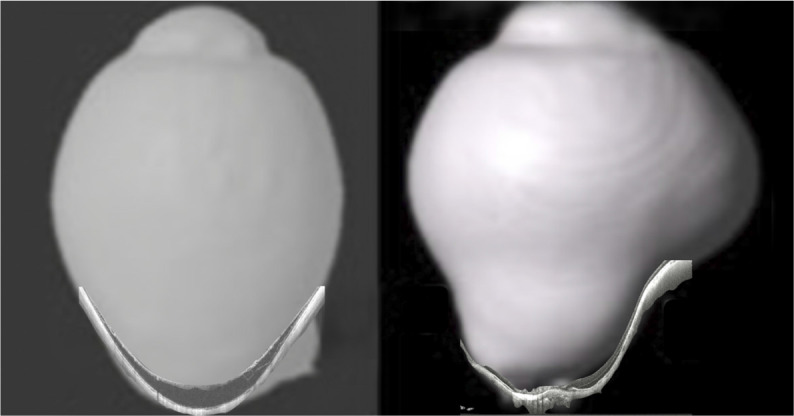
3D magnetic resonance imaging (MRI) of two eyes affected by posterior and lateral staphyloma, elongation of the eyewall and high myopia. The B-scan widefield OCT is superimposed on the posterior pole of the MRI images. The patient on the left has myopic traction maculopathy stage 2a, and the patient on the right has high myopia without traction.
